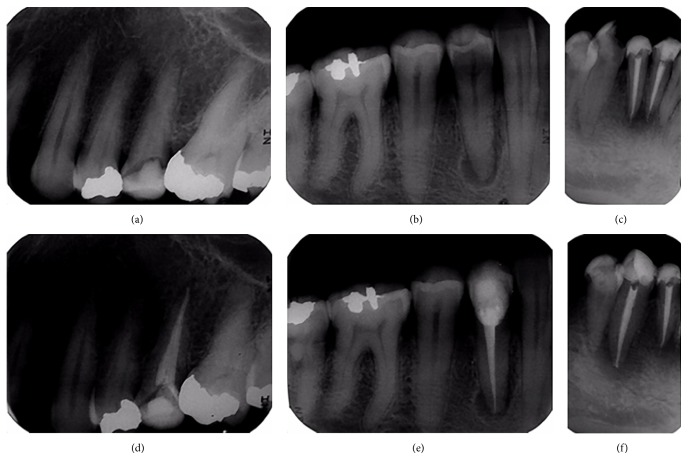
Digitized images of preoperative (a, b, c) and postoperative (d, e, f) conventional periapical radiographs using the MD300 USB X-ray Reader.
